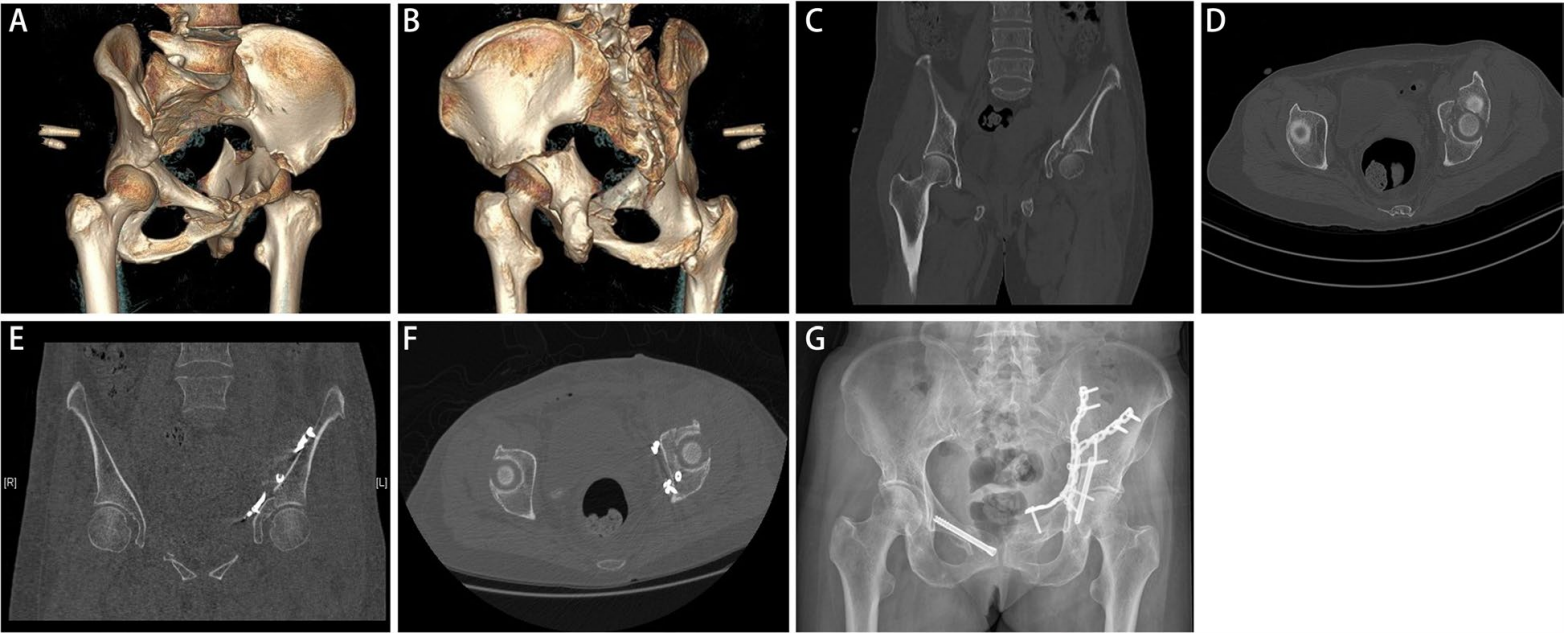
Preoperative and postoperative imaging evaluation. A-D Preoperative CT 3D reconstruction and scans of transverse acetabular fracture. E, F Postoperative CT scans showed the anatomical reduction. G Postoperative anteroposterior radiograph
